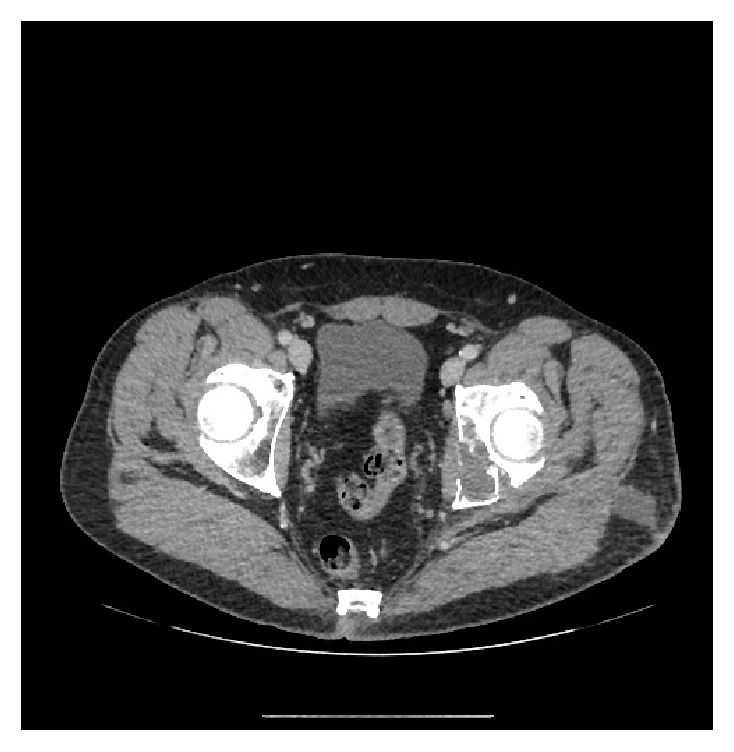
CT-scan: osteolytic lesion on the ischium.
